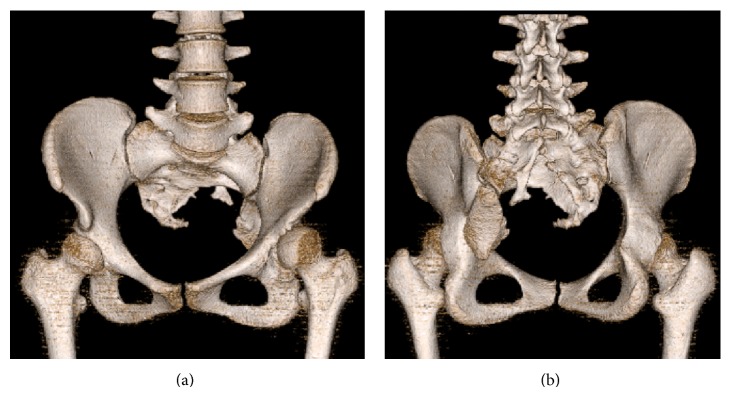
Pelvic three-dimensional CT reconstruction images demonstrated partial left sacral agenesis, in which a rudimentary sacrum had fused with the ilia below the sacroiliac joint. (a) AP view. (b) PA view.
